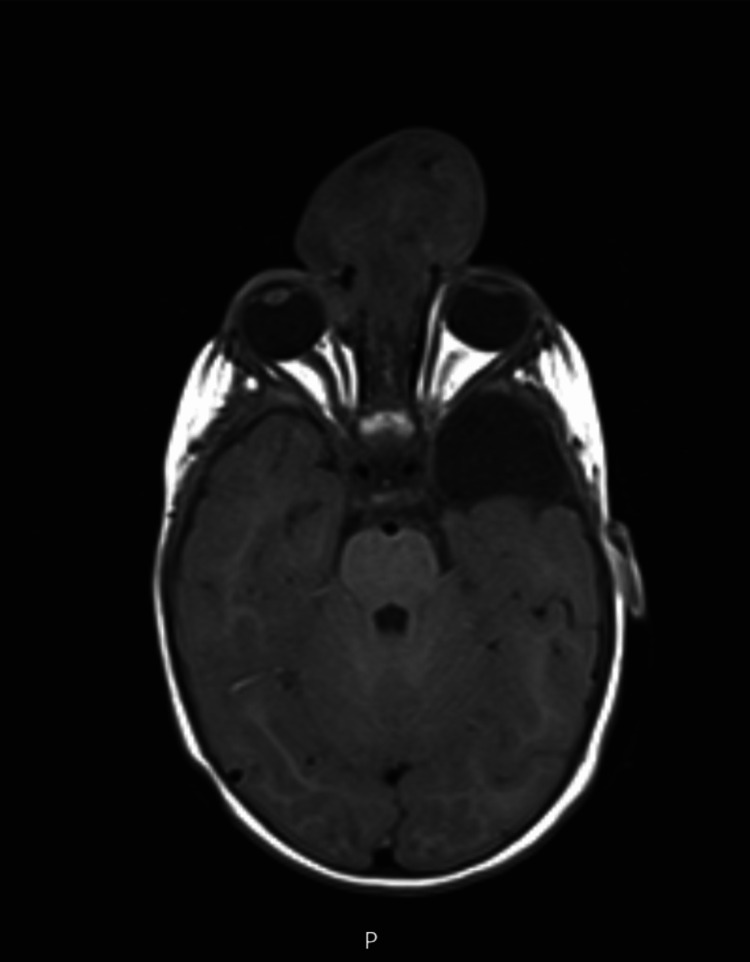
T1-weighted MRI of the frontoethmoidal encephalocele showing herniation of meninges and brain parenchyma through a bone defect and a meningoencephalic herniation into the right extraconical compartment.
